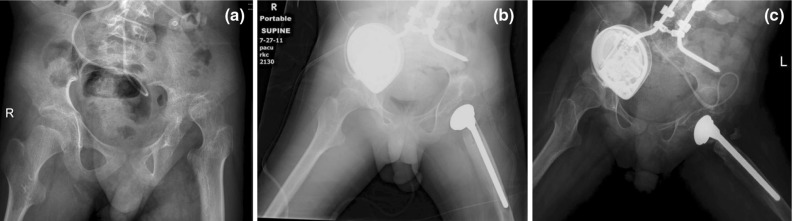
a Pre-operative radiograph of a 10-year-old child with cerebral palsy and a left painful, spastic dislocated hip. b Immediate post-operative radiograph. c Final radiograph at 48 months post-operatively of a child who had an excellent outcome with regards to pain, range of motion (ROM), and overall outcome
